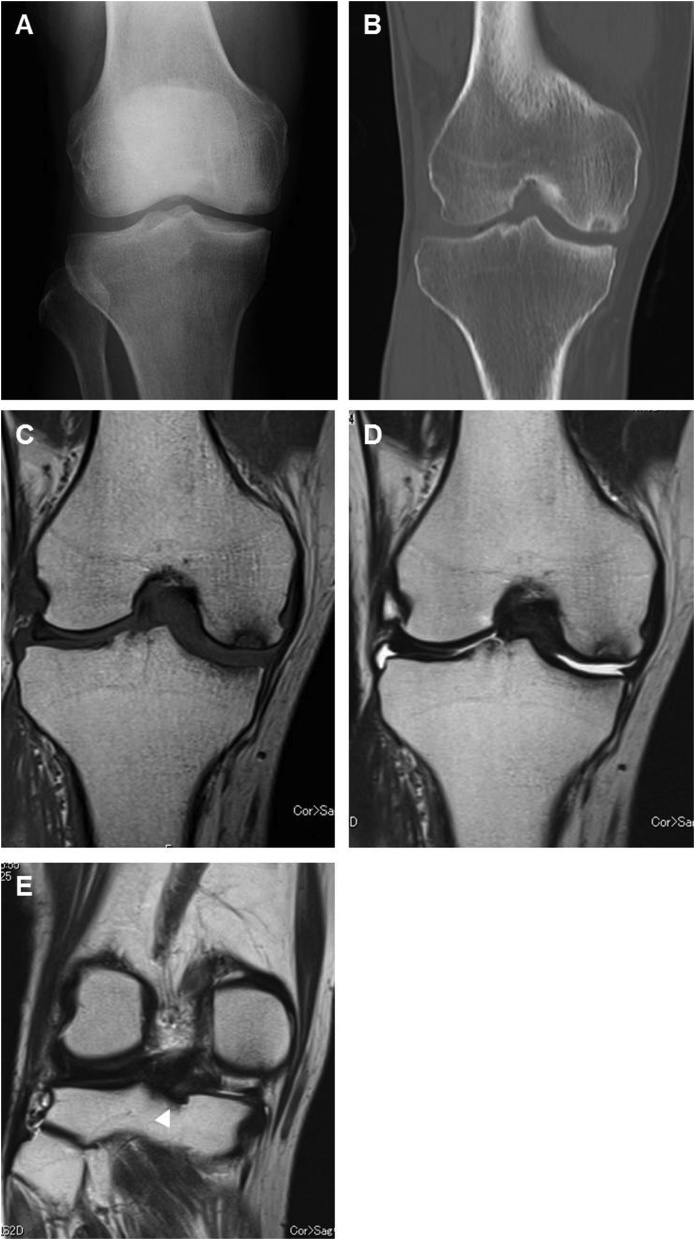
(A) AP radiograph 10 months after arthroscopic surgery showed focal radiolucency in the weight-bearing area of the medial femoral condyle. (B) Multiplanar reconstruction indicated a sclerotic band in the weight-bearing area of the medial femoral condyle. (C) The T1-weighted image showed diffuse low signal intensity in the medial femoral condyle, and an associated band of lower signal intensity was seen in the area of the lesion. (D) The T2-weighted image had an inhomogeneous area of high signal intensity in the corresponding region and showed a focal area of low signal intensity underlying the articular cartilage. (E) T2-weighted images generally showed that the posterior root of the medial meniscus had been preserved (arrowhead).
